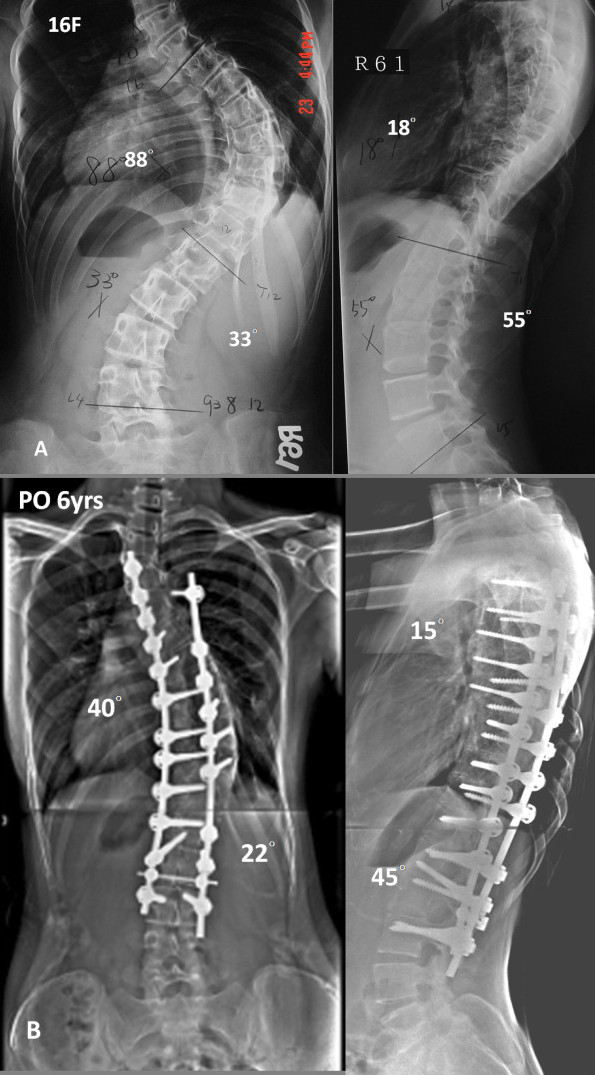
A Preoperative AP, and lateral radiographs of a 13 year-old-female with 1A curve. B, The patient underwent anterior fusion followed by posterior spinal fusion from T3 to L3 with all-screw construct. Postoperative radiographs of 6 years followup showed 55% correction rate.
